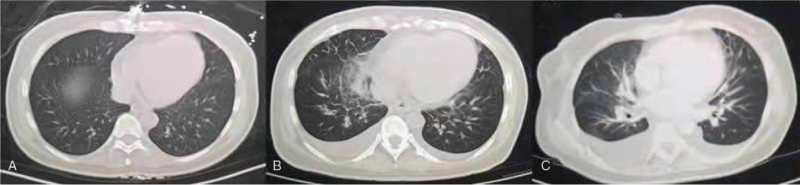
Dynamic changes of chest CT examination. (A) Chest CT examination at 8:00 am on November 17, 2021 revealed no significant lung abnormalities. (B) Chest CT examination at 5:39 am on November 22, 2021 revealed pulmonary edema in both lungs and a small pleural effusion. (C) CT re-examination at 11:30 am on November 22, 2021 showed progressive pulmonary edema, atelectasis of the right lower lung. CT = computed tomography.
